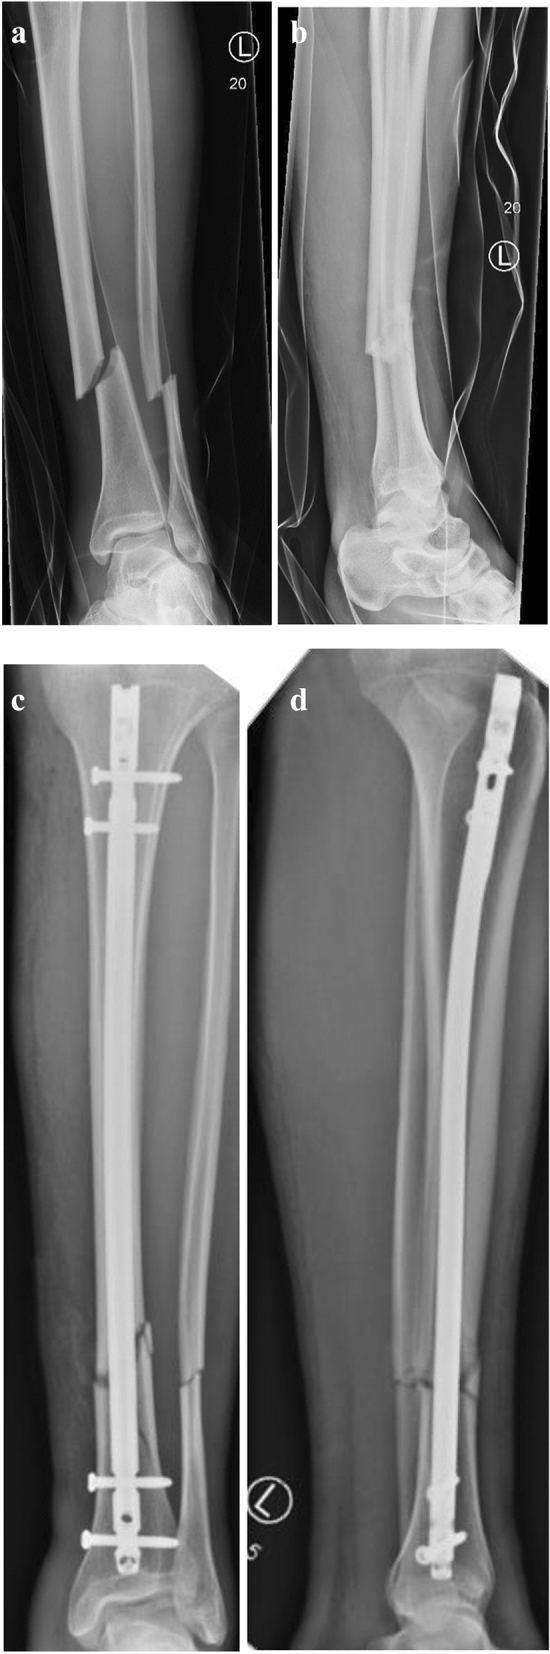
Thirty-two-year-old male patient with a closed fracture of the lower leg (AO 42B2) X-ray in ap (a) and lateral view (b). AP X-ray (c) and lateral (d) view after closed reduction and internal fixation via intramedullary nail (Tibia EXPERT™ nail, Synthes, West Chester, PA, USA)
